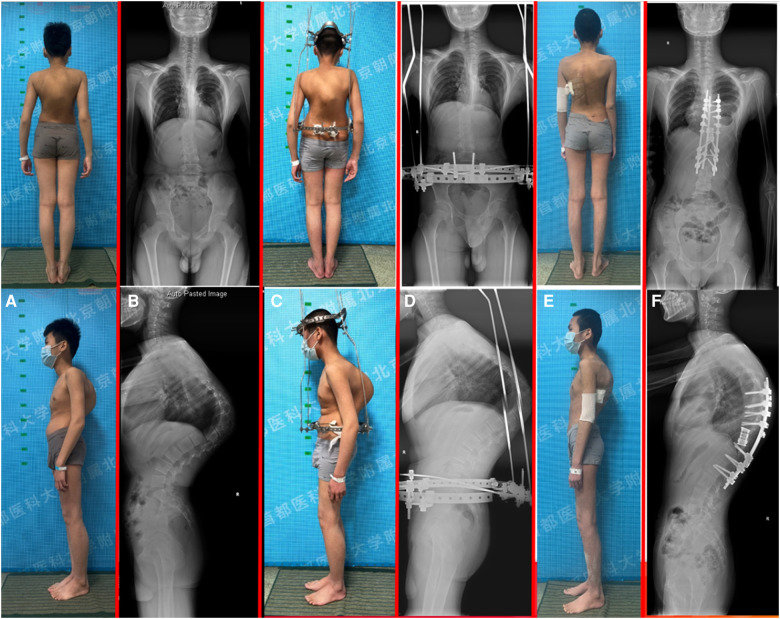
A 13-year-old male with progressive kyphosis and massive osteolysis in the thoracic vertebrae. (A) patient's preoperative appearance; (B) preoperative standing whole-spine radiograph demonstrated a thoracic kyphosis curve of 122°; (C) patient's halo-pelvic traction appearance; (D) standing whole-spine radiograph after halo-pelvic traction; (E) patient's postoperative appearance; (F) postoperative standing whole-spine radiograph demonstrated the thoracic kyphosis was reduced to 48°, with a correction rate of 60.8%.
